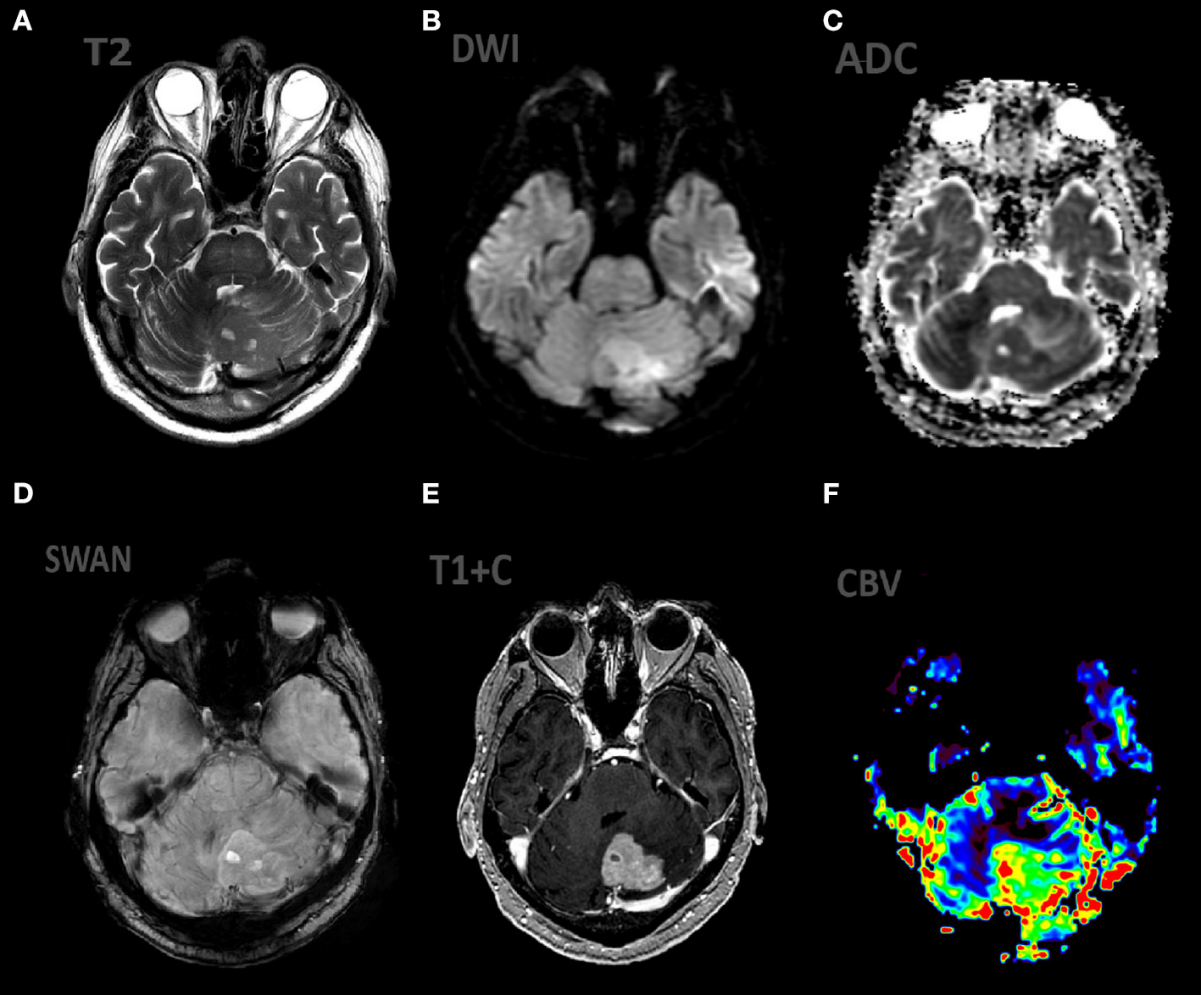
Patient V., male, 66 y.o. presented with gate disturbances. MRI ax: (A) T2WI; 6, (B) DWI, (C) ADC, (D) SWAN, (E) T1 + C, (F) DSC-PWI. Axial Brain MRI: there is lesion in periventricular white matter, the lesion involve left cerebellar peduncle, hemisphere. The lesion demonstrates high SI on T2WI, restricted diffusion (with low ADC values), homogeneous contrast enhancement, elevated rCBV values.
